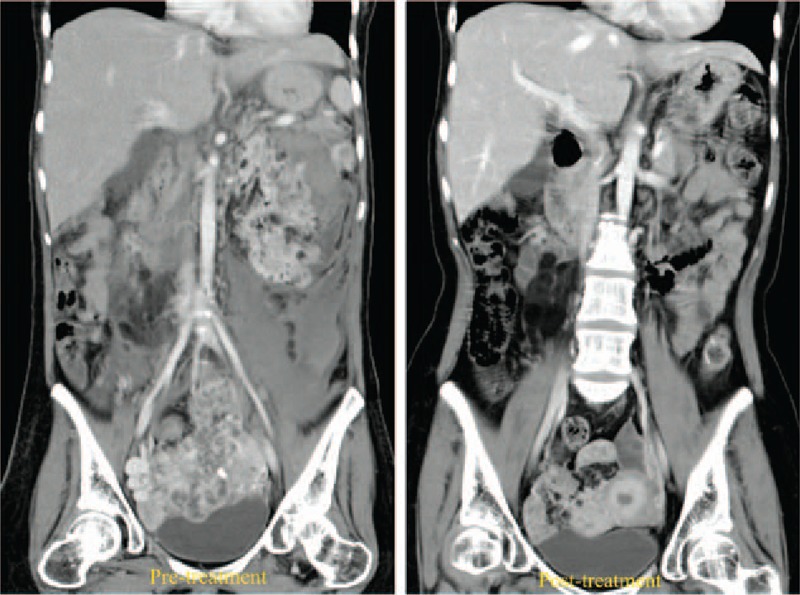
Contrast-enhanced abdominal CT images of the first patient before and after 4 months of everolimus 10 mg/ day treatment. Pretreatment image shows ruptured left AML and a large pelvic mass. Post-treatment, significant regression was seen in both renal and pelvic tumors. AML = angiomyolipomas, CT = computed tomography.
